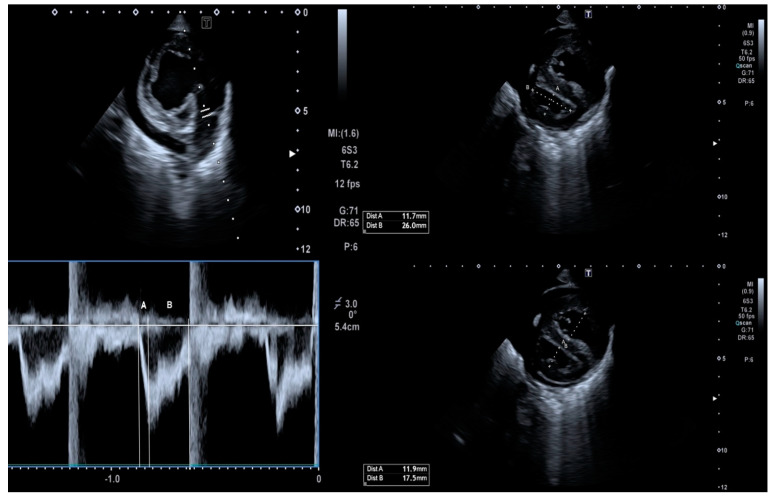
This figure shows the semiquantitative echocardiographic parameters used to assess the pulmonary artery pressures in this study. In a short axis view, we interrogated the RV outflow tract flow with pulsed-wave Doppler (left-sided image) and calculated from the resultant spectral Doppler imaging the ATET ratio (A/B). We also calculated the systolic LVEI at the level of the papillary muscles (top right-sided image) as the ratio between mediolateral and anteroposterior diameters of the LV (A/B). Finally, we calculated the RVLV ratio at the same level (B/A).
